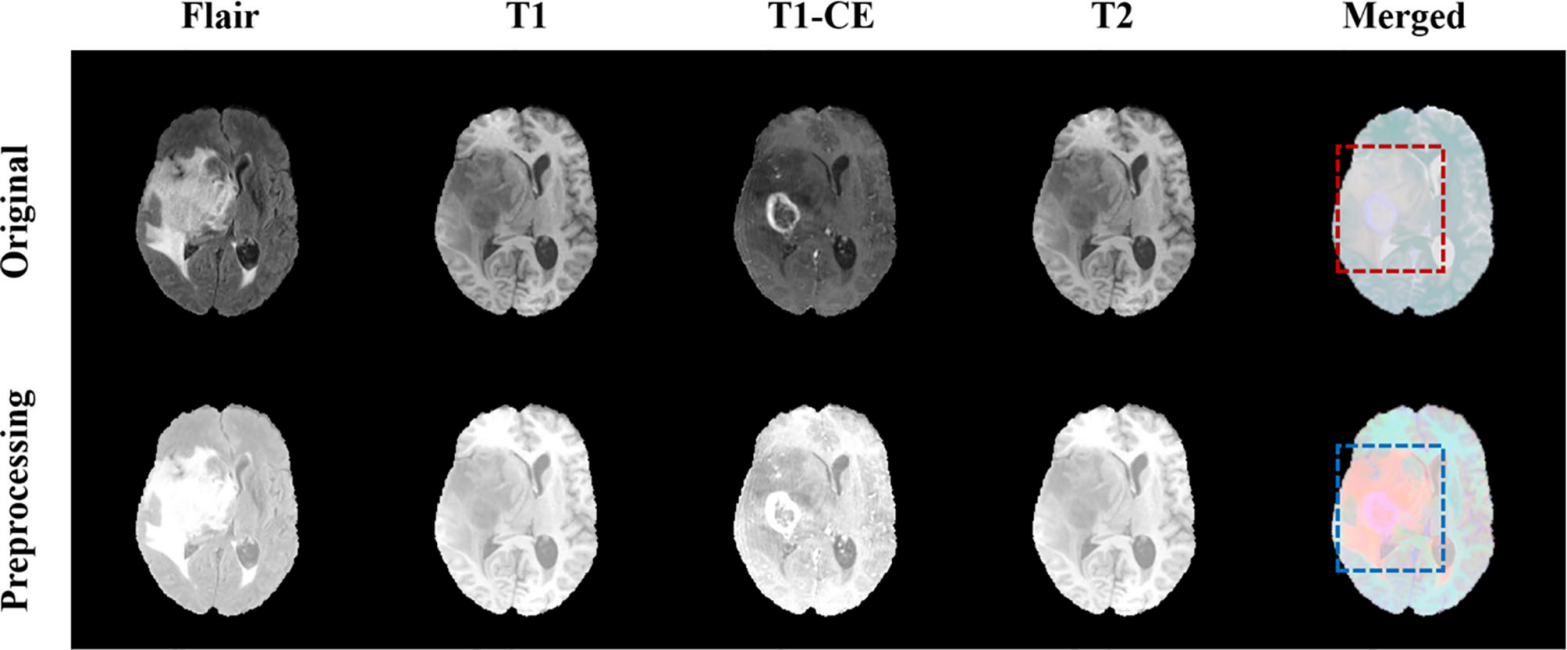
Comparison of MRI before and after pretreatment. The first line is the original image, the second line is the preprocessed image. The first four columns are the images of the four modalities Flair, T1, T1-CE, and T2, and the last column is the merged image.
